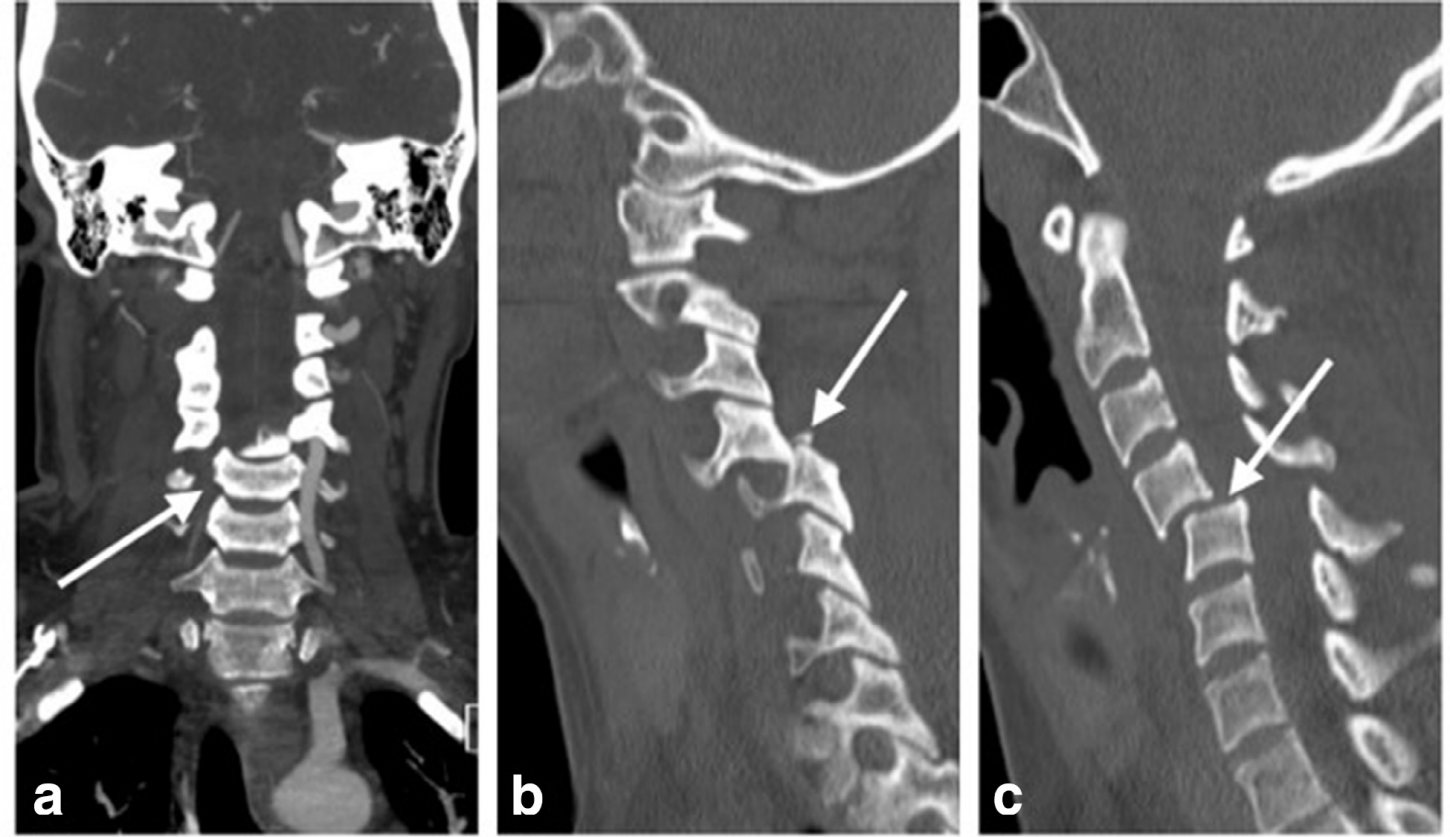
31-year-old female who sustained a blow to the head. a coronal CTA image demonstrating a ringt vertebral artery dissection (arrow). This extended form the level of the C3/4 interspace to the C6 vertebral body. b. Sagittal reconstruction with done displaced anterior to the superior facet of the C5 vertebral body,in keeping with a jumped/locked facet joint. A mildly displaced tiny fracture fragment is also demonstrated at the tip of the superior facet of the C5 vertebral body (arrow). c. Sagittal reconstruction with bone windows at the level of the midline demonstrates grade 2 anterolisthesis of C4 over C5 (arrow).
